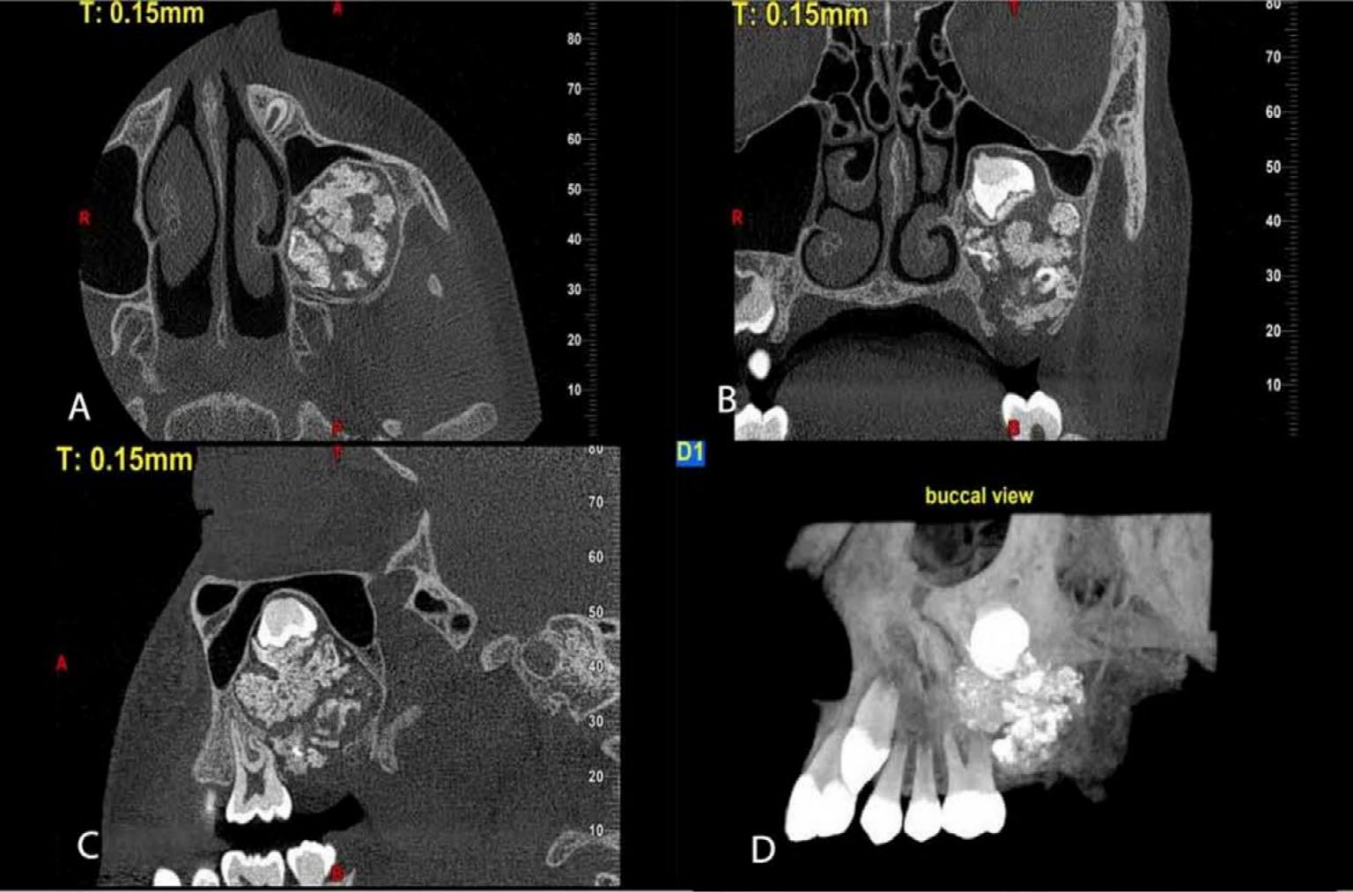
Preoperative CBCT. (A) axial view, (B) coronal view, (C) sagittal view, (D) reconstructed view (MIP).
